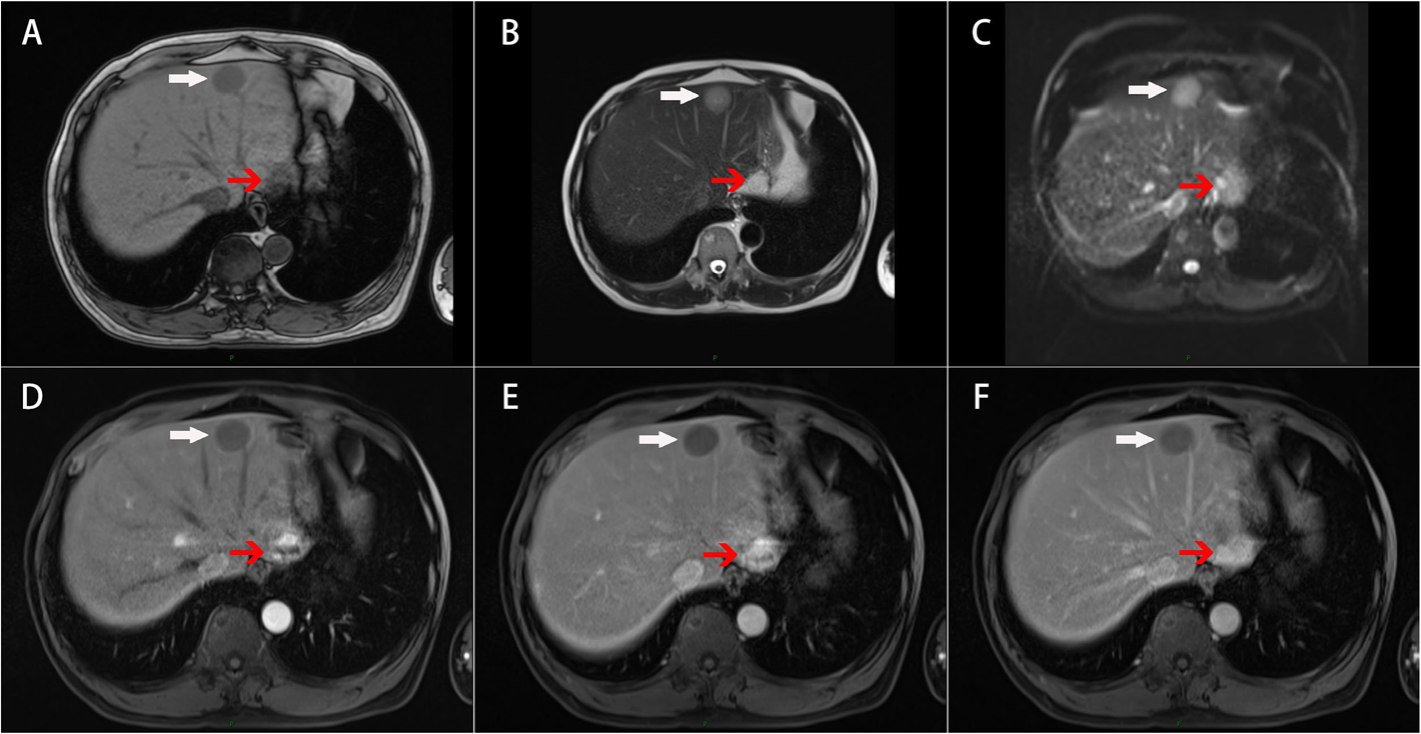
Enhanced MRI scans. a Hypointense on T1-weighted images (white arrow). b Hyperintense on T2-weighted images. c DWI showed mild limitation of dispersion. d Ring-shaped enhancement. e Portal vein stage, slight ring-shaped enhancement. f Delayed stage, decreased enhancement. Cavernous hemangioma (red arrow)
